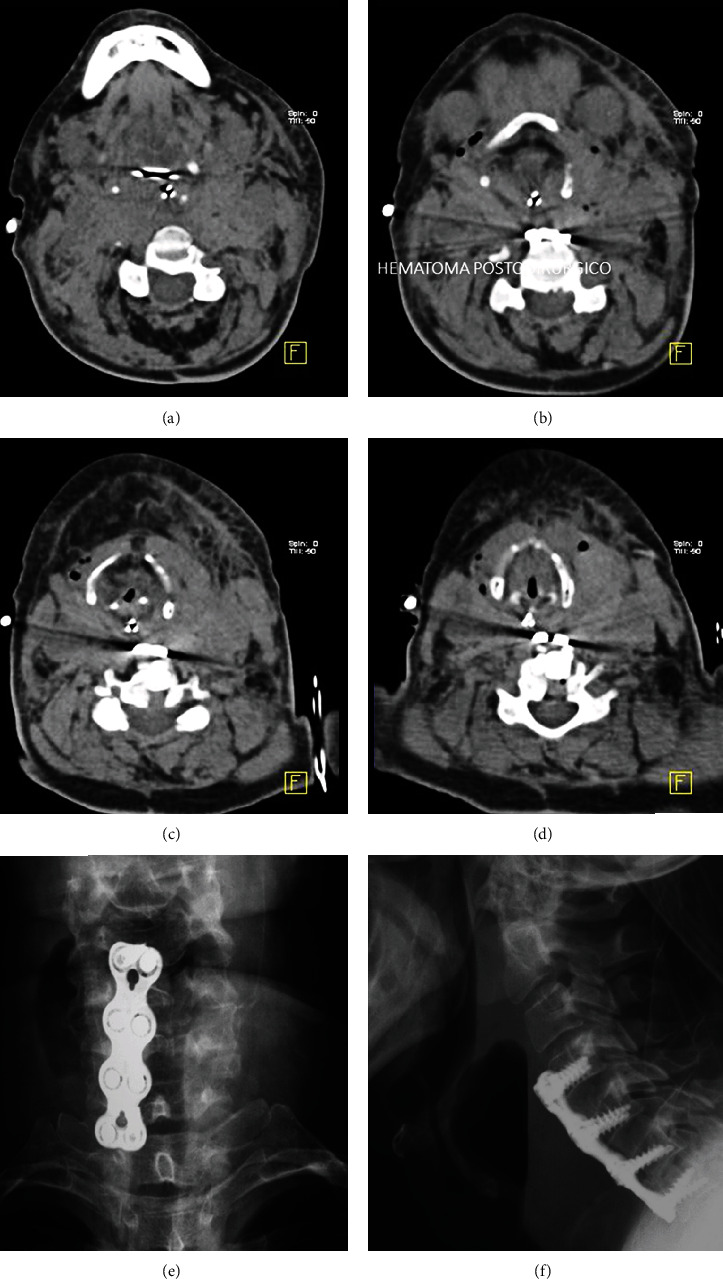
(a–d) CT of the cervical spine. Axial view of hematomas in the anterior aspect of the cervical spine. (e, f) Anterior and lateral views of the cervical spine showing hematomas anterior to a cervical plate.
